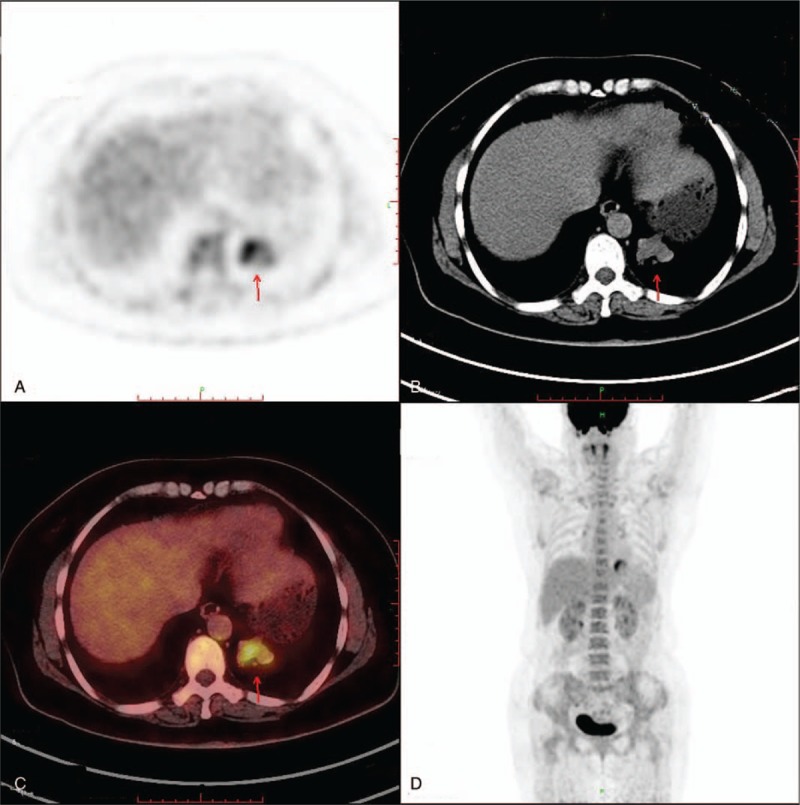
A computed tomography (CT) scan of the chest revealed a 3.0 × 3.0 cm left lung mass. Maximum intensity projection PET (D) and axial (B) CT, corresponding PET (A), and fused (C) images showed FDG uptake of the lesion (arrow) with SUVmax of 6.5.
